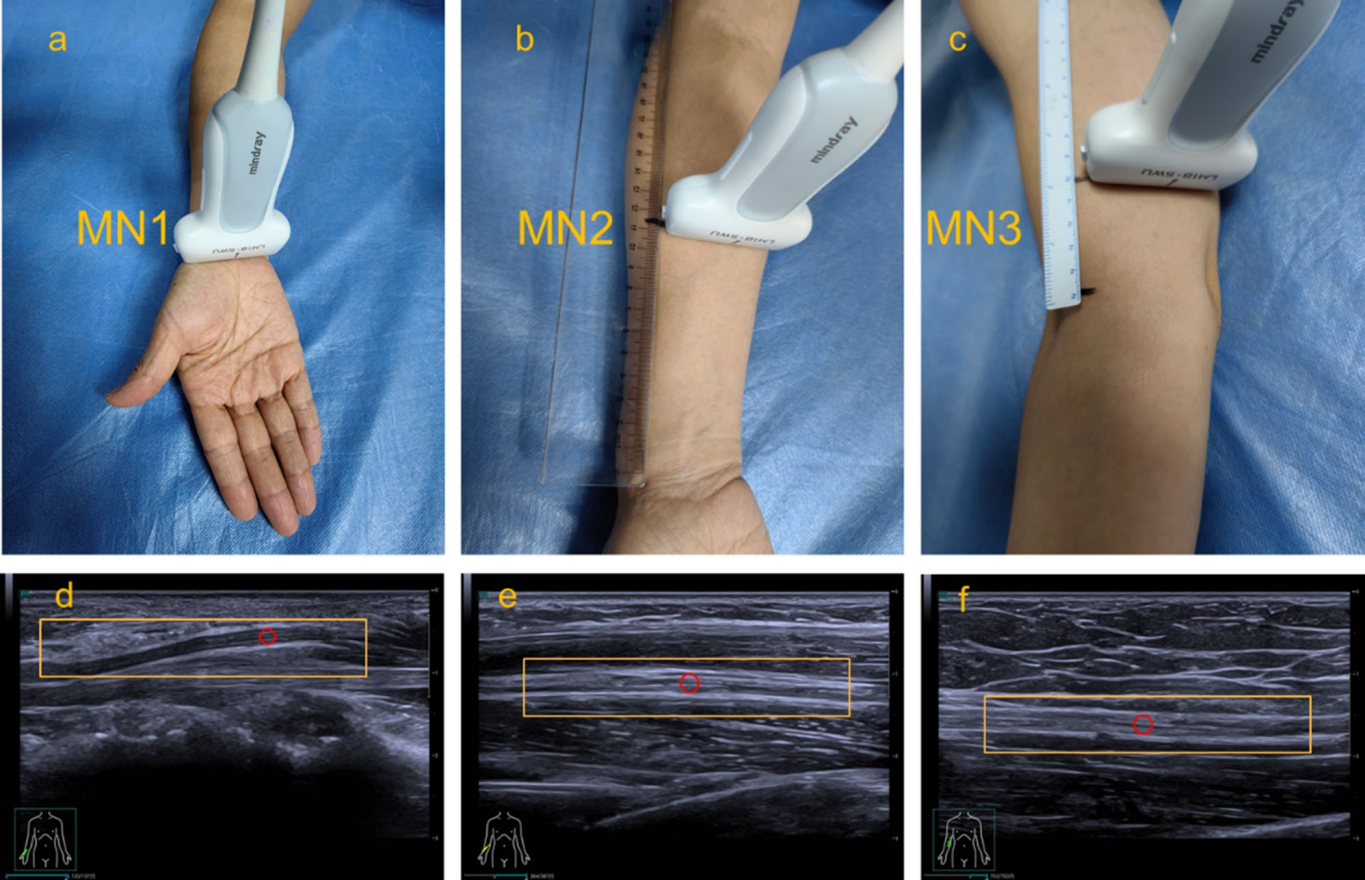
Representative probe placement and corresponding STVi measurements of the MN at three anatomical sites in a healthy volunteer. (a–c) Probe positioning for MN1, MN2, and MN3, respectively. (d–f) Corresponding longitudinal grayscale ultrasound images with the region of interest (ROI) indicated by the rectangular box and circular Q-box placed within the nerve for viscosity and stiffness quantification
